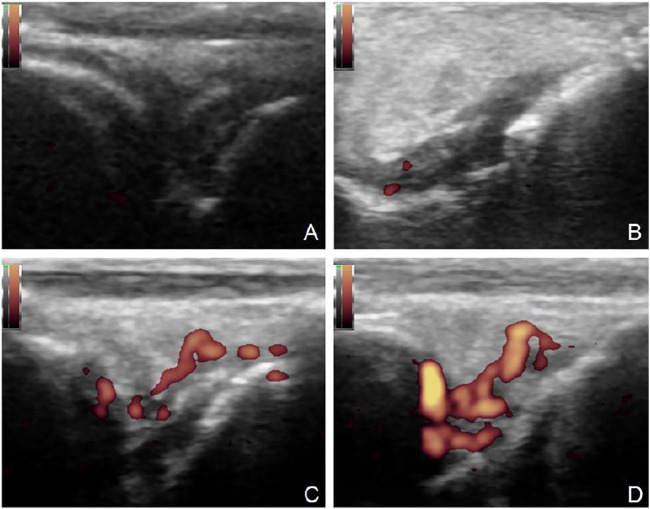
Power Doppler ultrasound of synovitis. Score 0: no color blood flow signal (TP-SLN group, (A). Score 1: dot color blood flow signals (TP group, (B)). Score 2: short linear color blood flow signal with distribution less than 1/2 of synovial area (DMSO group, (C)). Score 3: reticular blood flow signals distributed more than 1/2 of synovial area (control group, (D)).
